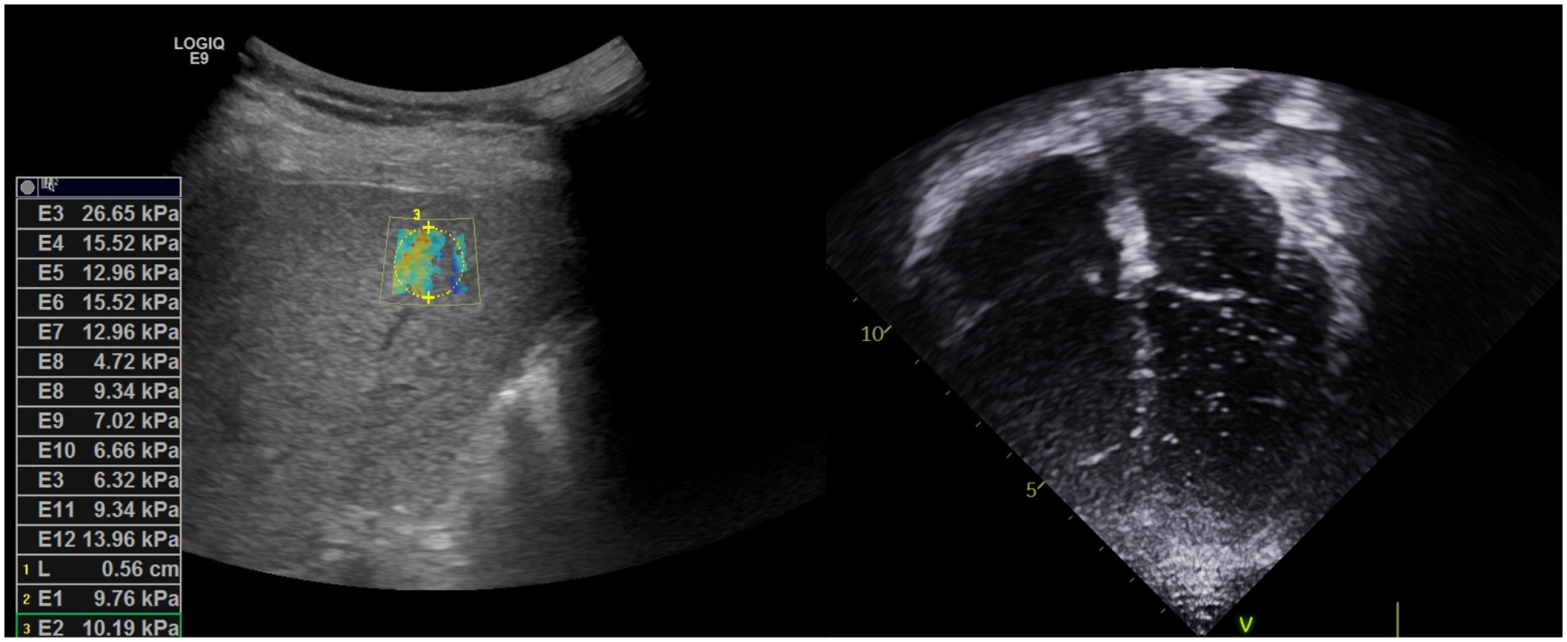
Left: Liver elastography revealed slightly coarse and increased liver parenchymal echogenicity, and increased liver stiffness index. Right: Contrast-enhanced echocardiography with agitated saline revealed microbubbles appearing in the left ventricle after three cardiac cycles following the appearance of bubbles in the right ventricle, indicating the presence of right to left intrapulmonary shunt.
